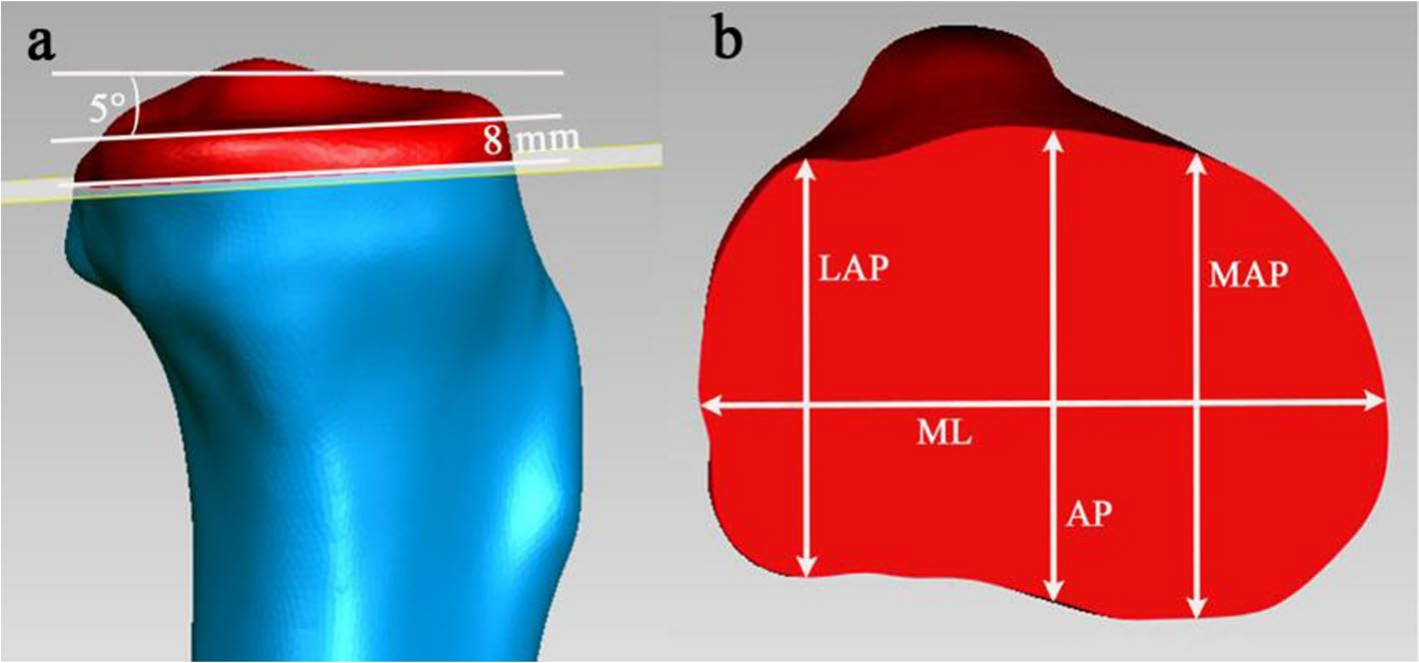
Proximal tibia resection and measurement on CT images. a Resection method of proximal tibia. b Measurements of resected tibial surfaces
